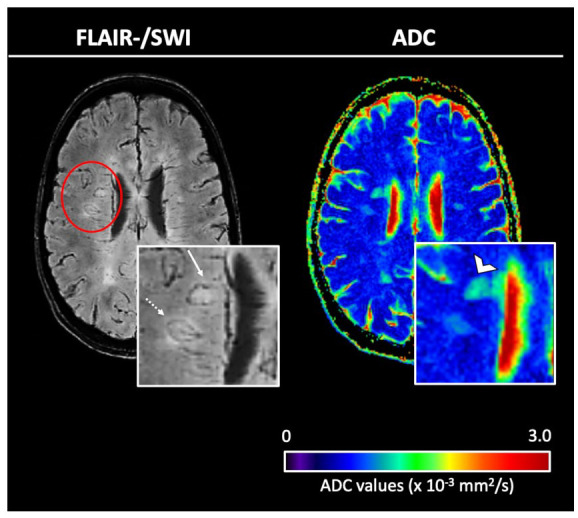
Left: co-registered, overlaid fluid-attenuated inversion recovery (FLAIR)/susceptibility-weighted images (SWI), right: quantitative maps of the apparent diffusion coefficient (ADC). FLAIR/SWI demonstrates representative examples of two iron rim lesions (IRLs); one periventricular IRL (arrow) and one deep white matter IRL (dotted arrow). Note the higher ADC values in the periventricular lesion (open arrowhead) compared to the lesion localized in the deep white matter.
